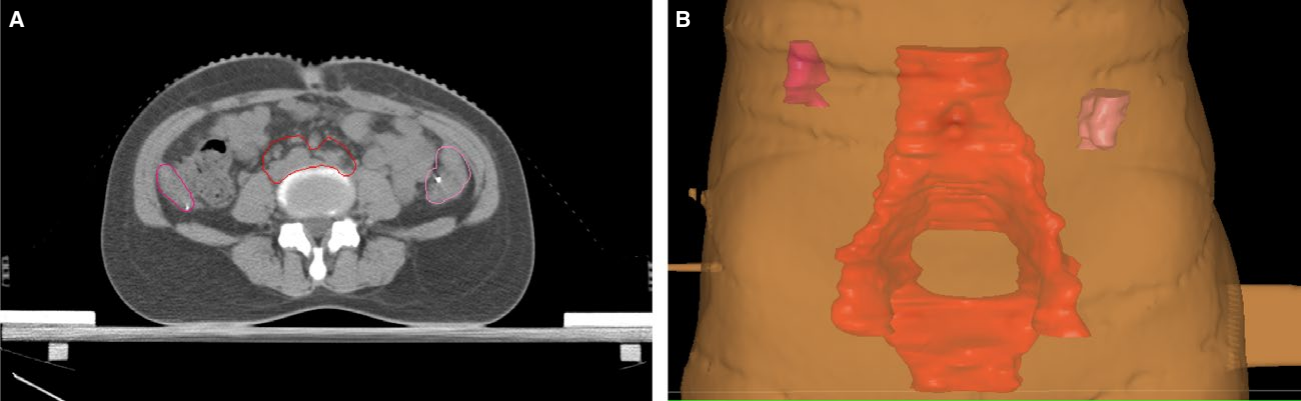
Ovary position: (A) ovary position in CT image; (B) reconstruction of ovary position in IMRT plan system. Red region is the patient's clinical target volume (CTV). IMRT, intensity‐modulated radiation therapy.
